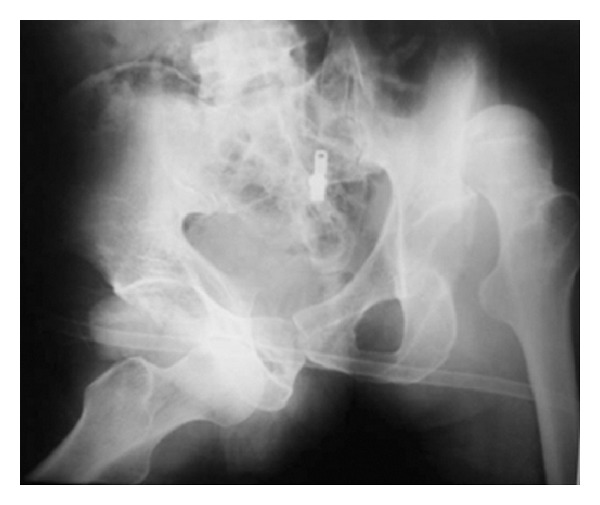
Radiograph showing anterior dislocation of right hip and posterior dislocation of left hip joint, without any associated fracture.
